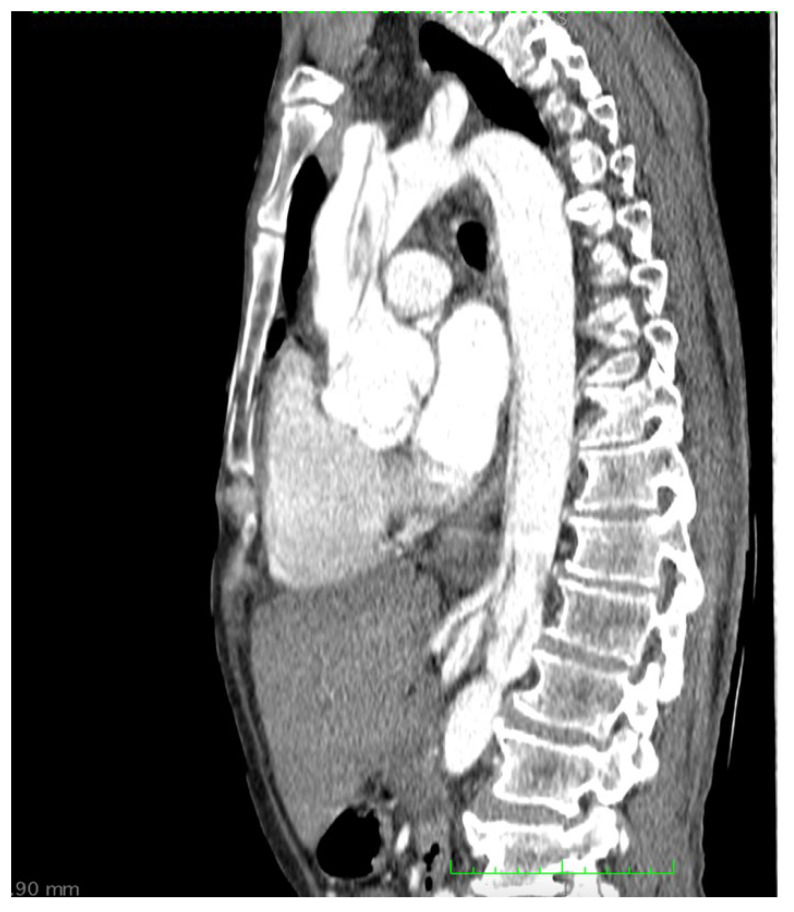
CT showing intimal flap at the level of the ascending aorta, aortic arch, thoracic and abdominal aortas and superior mesenteric artery. Celiac trunk with origin in a small true aortic lumen.
